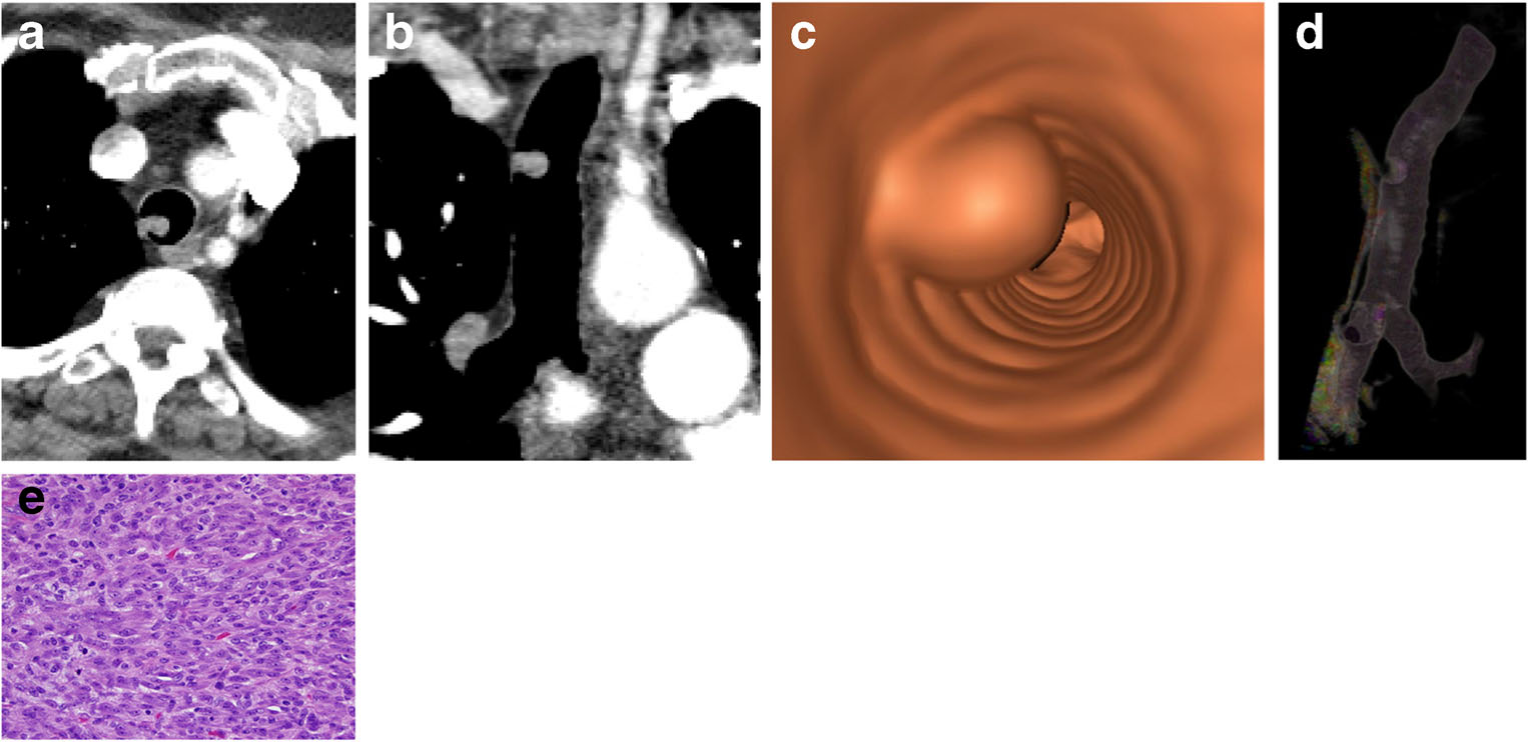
Seventy-two-year-old woman with persistent cough and bloody sputum. a Axial and b coronal CT demonstrates a polypoid soft tissue mass. c, d Virtual bronchoscopy and 3D VR image of the mass. e Pathology reveals a spindle cell proliferation with mild to moderate nuclear pleomorphism compatible with atypical spindle cell carcinoma
